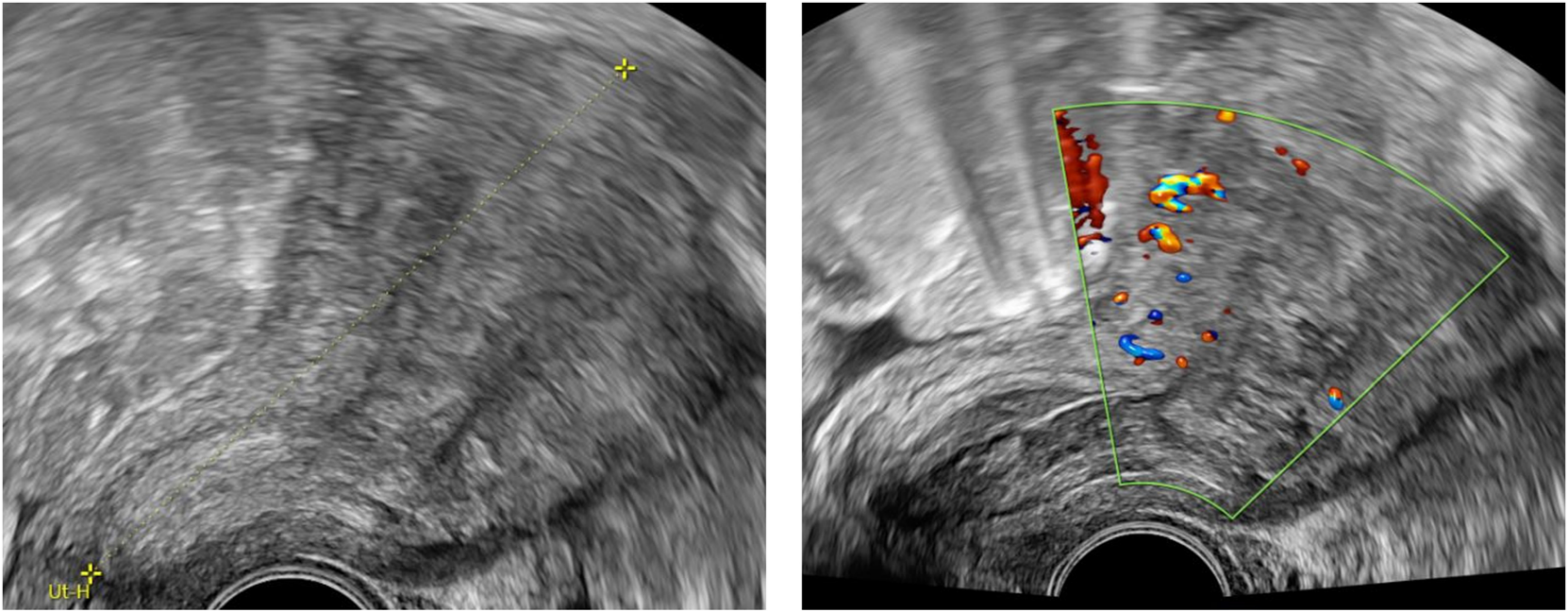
Ultrasound findings four weeks after delivery. The ultrasound findings four weeks after delivery revealed a normal sized uterus with a small 1 × 2 × 3 cm intrauterine diffusely perfused inhomogenous structure.
